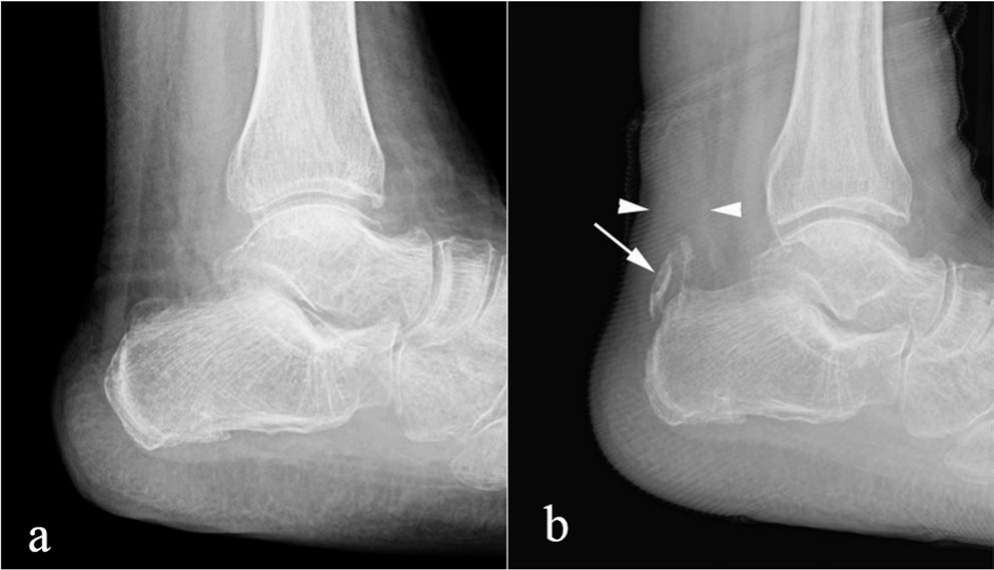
Type III calcaneal tuberosity fracture. 53-year-old woman initially presented after a slip and fall with a distal fibula fracture (not shown). Lateral radiograph (a) shows normal calcaneal tuberosity. Seven weeks later (b), the patient fell and complained of 8/10 heel pain. Lateral radiograph shows a calcaneal avulsion fracture (arrow in b) and thickening of calcaneal tendon shadow (between arrowheads in b). No further follow up images were obtained
